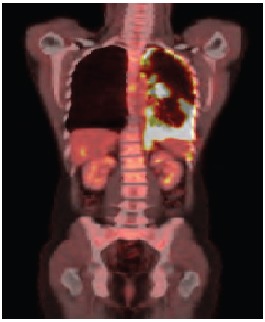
PET–CT showing evidence of the presence of pleural thickening and increase of radiopharmaceutical capture.
